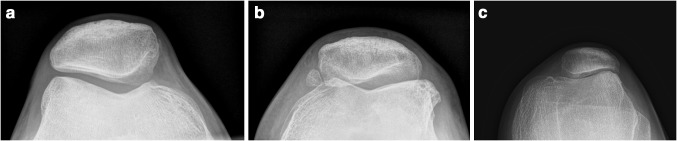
Conventional radiographs of the three grades of patellofemoral OA according to the Iwano classification showing a grade 0, no features of OA; b grade I, remodelling; c grade II, joint space narrowing of <3 mm
